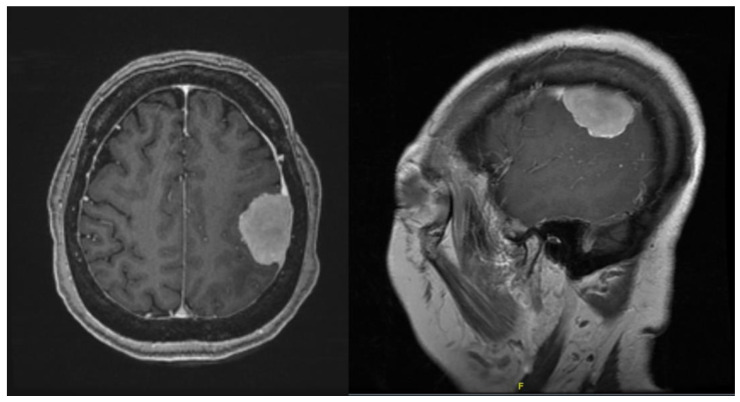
Contrasted T1 MRI showing homogenously enhancing frontal convexity meningioma.
